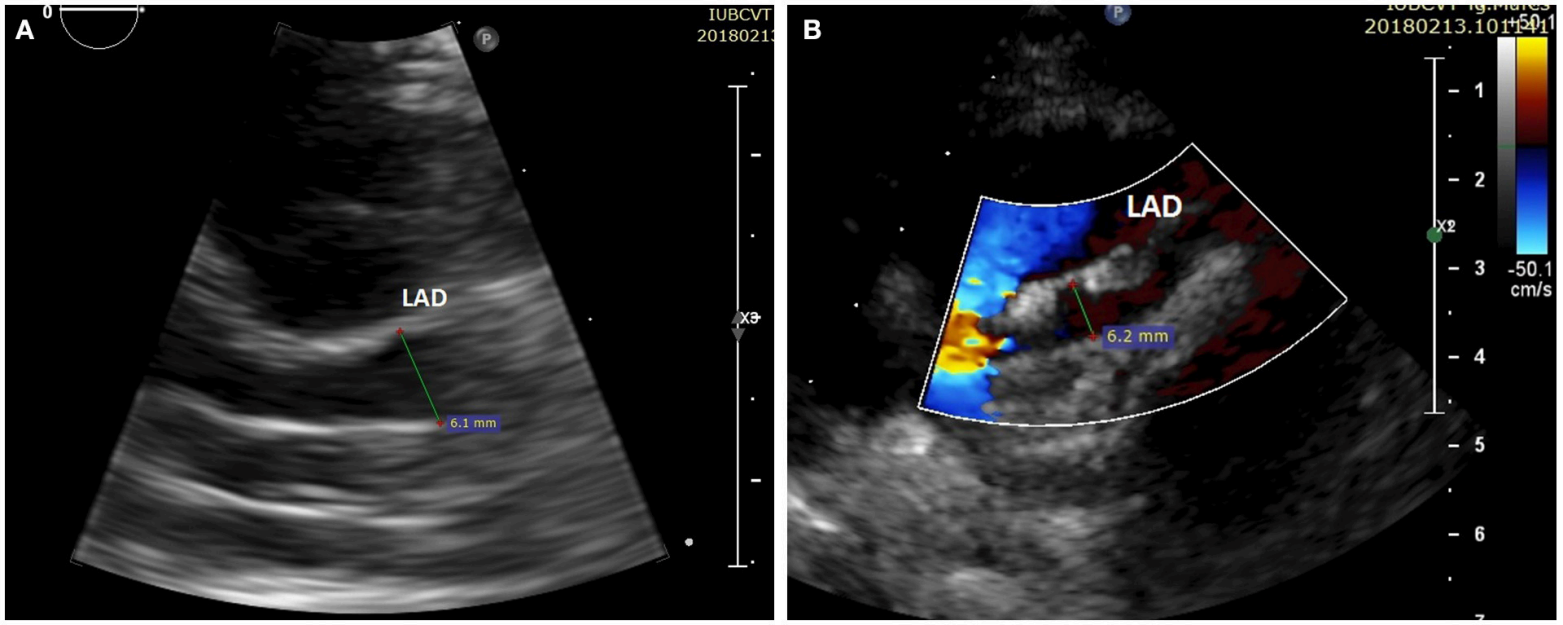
(A) Cross-sectional view at the level of the great arteries: left anterior descending (LAD) with an aneurysm (diameter > 6 mm, Z score + 16.45). (B) The same image as 3A with color Doppler.
